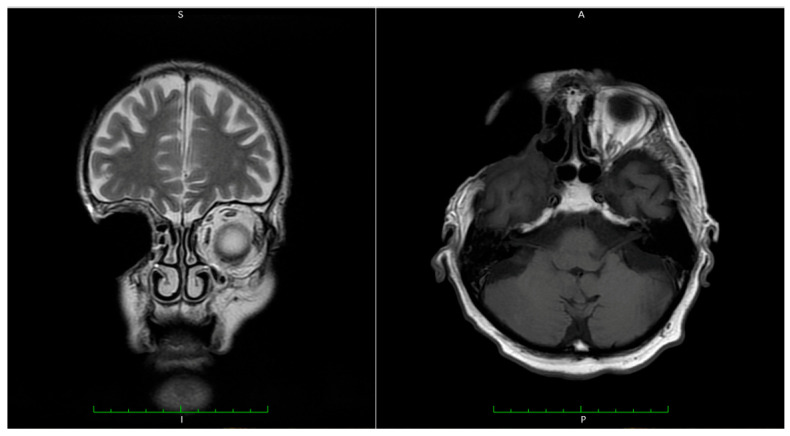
Magnetic resonance imaging (MRI) of the patient, 6 months postoperatively, with no local recurrence.
